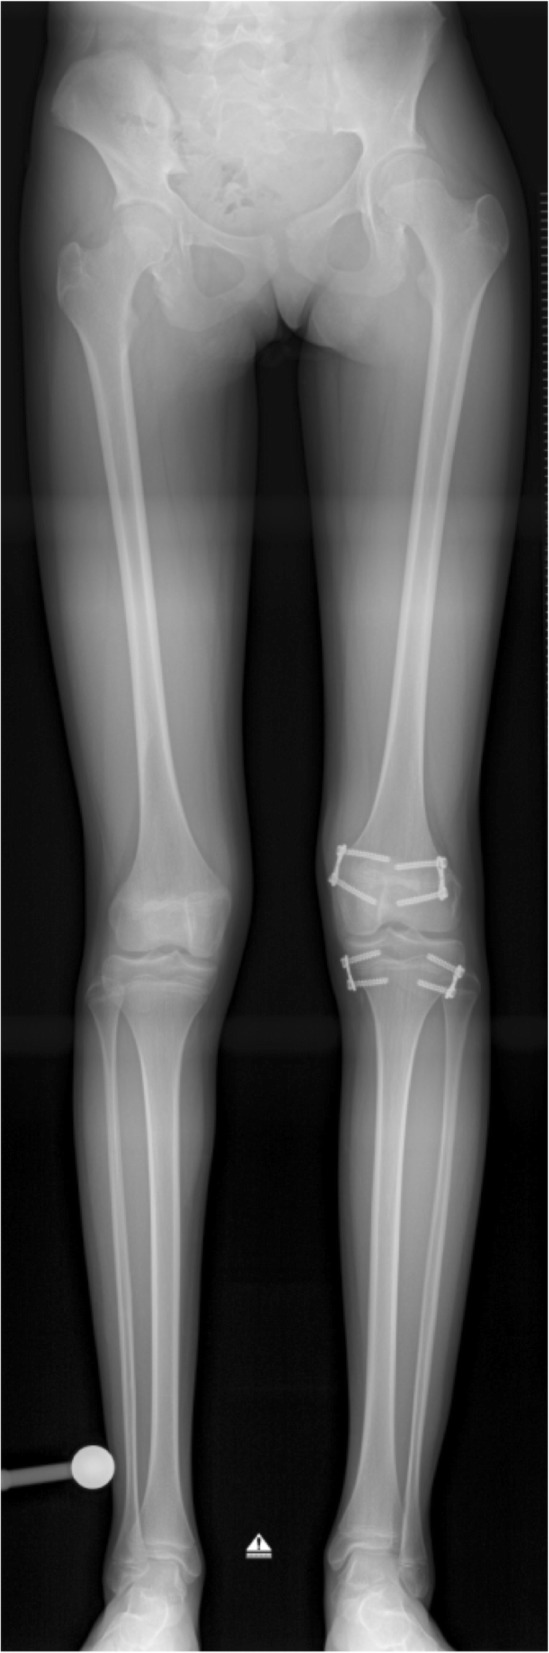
Long-standing X-ray of a patient with limb length discrepancy. Temporary epiphysiodesis with eight-plates (TE) was performed as a tension band procedure with one plate at both the medial and lateral side of the epiphysis of the left distal femur and proximal tibia
